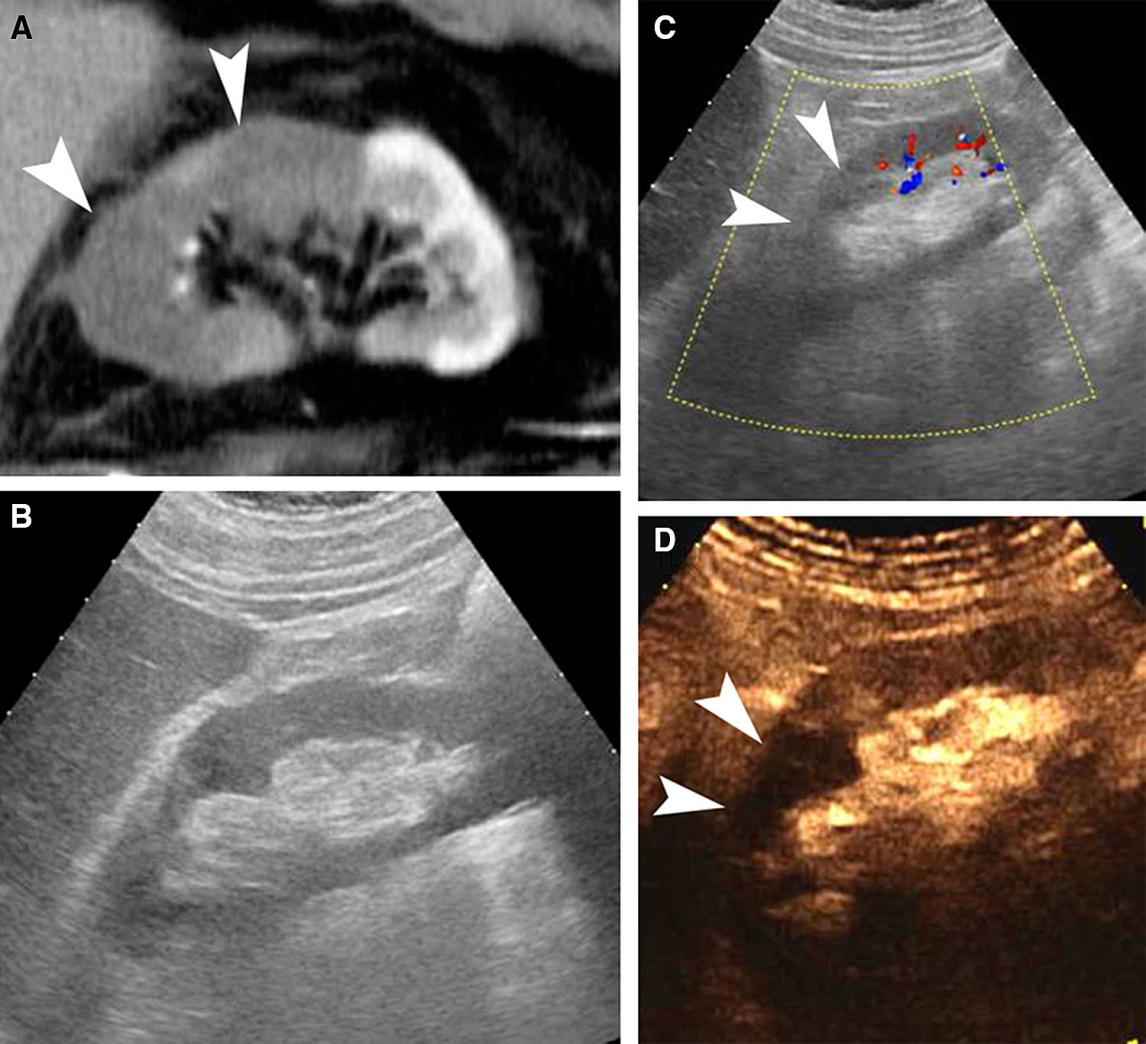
Imaging findings in a patient with renal infarction. Oblique sagittal MDCTA image (A) showing the kidney in long-axis, revealed the presence of an upper pole renal infarct (arrowheads). On follow-up US, long-axis B-mode technique (B) did not visualize any significant alterations in parenchymal echogenicity. Respective color Doppler technique image (C) demonstrated less blood flow signals on the upper half of the kidney (arrowheads), in keeping with the infarct. Respective CEUS image (D) readily confirmed the diagnosis of renal infarction (arrowheads)
